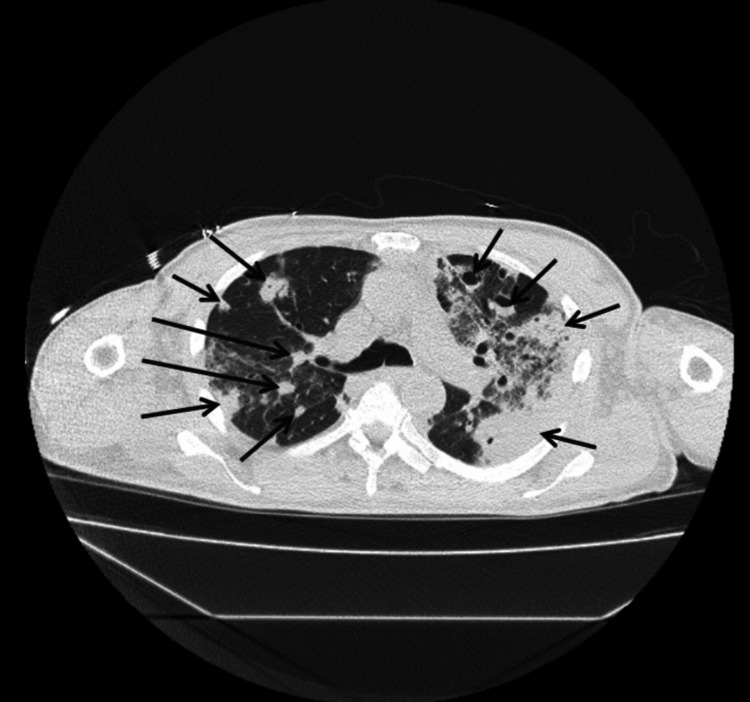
CT chest axial view without contrast showing upper-middle parts of both lungs. The arrows show multiple, bilateral diffuse airspace cavitations with air bronchograms in some cavities consistent with septic pulmonary emboli.
